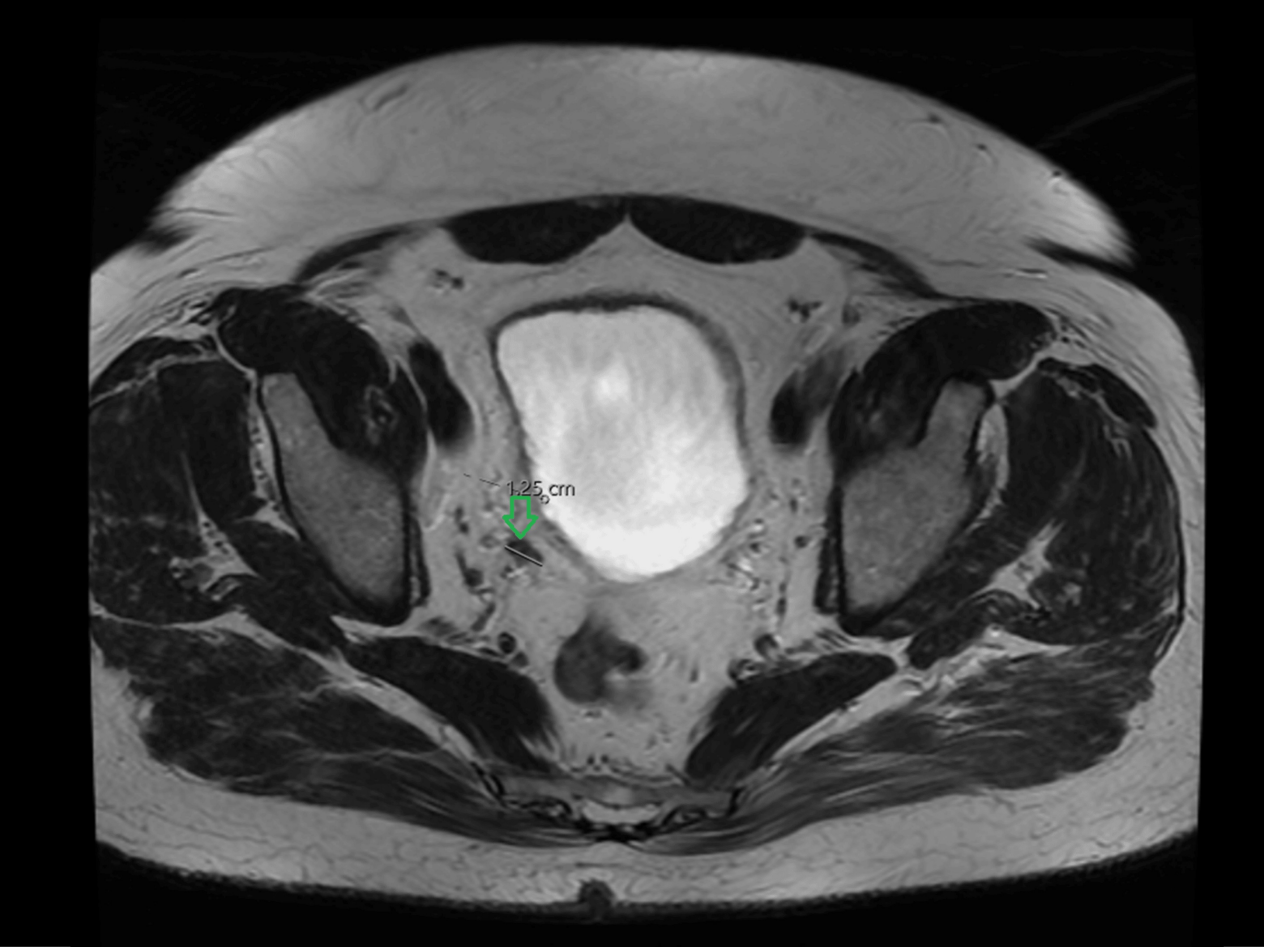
MRI Pelvis (March 2023) Axial T2-weighted image from 2023 showing the original right-sided soft tissue inflammation (green arrow), which was stable but persistent at surveillance, measuring 1.25 cm.
